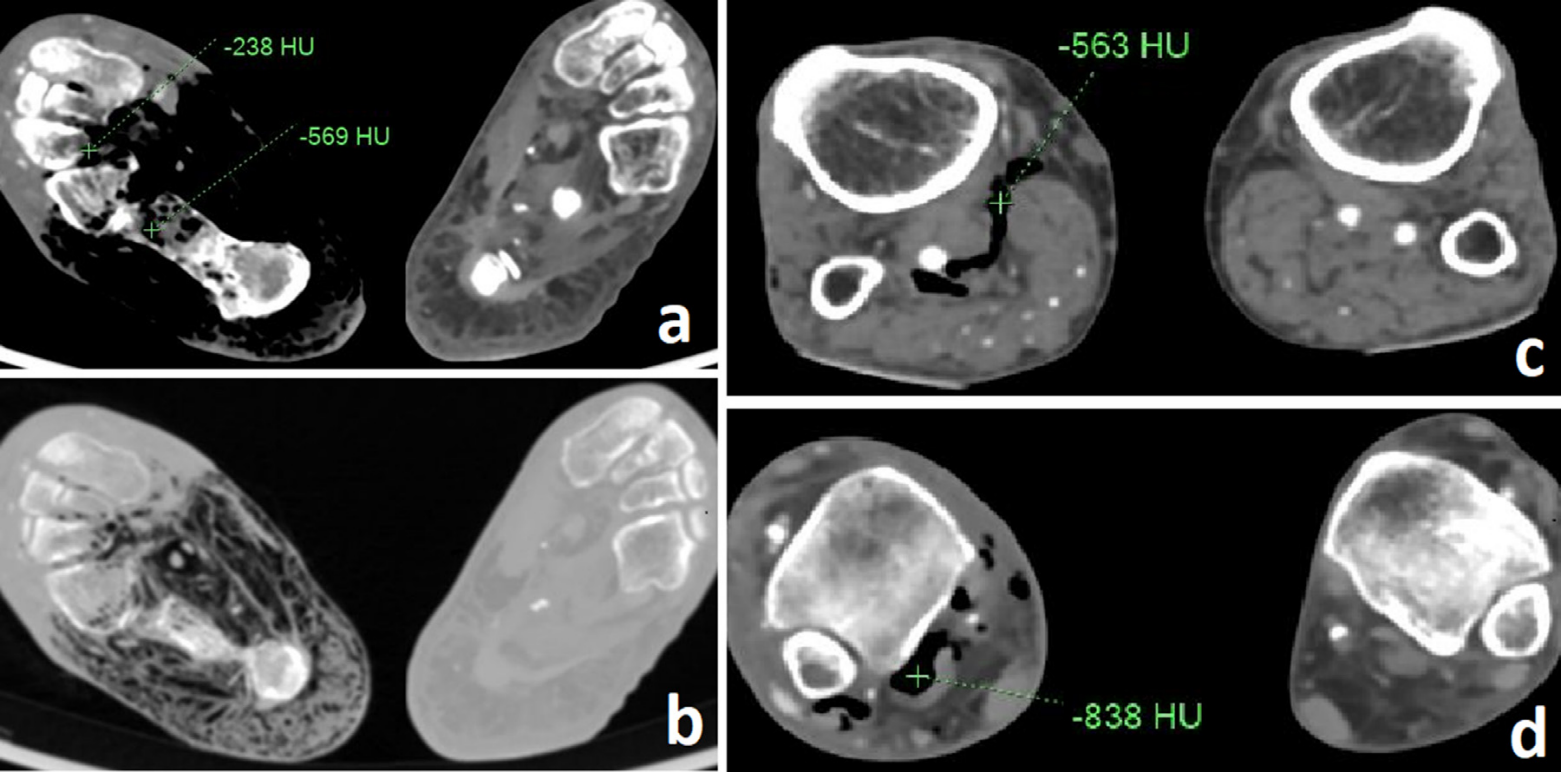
(A) Axial CT images of feet in soft tissue window of a 59-year-old male show cortical erosions and bone destruction in tarsal bones of right foot (calcaneus, cuboid and cuneiforms) with presence of intraosseous gas (indicated by Hounsfield units of air) and gross amount of gas in sub-cutaneous planes of right mid and hind foot. (B) Axial CT image in lung window supports the presence of air as black areas in the involved regions of the right foot. These features are suggestive of “Emphysematous osteomyelitis” involving tarsal bones of right foot with extension to soft tissues. (C and D) Axial CT angiogram images at the level just below knee (C) and at the level just above ankle (D) show presence of gas in deep tissue plane of right leg likely due to tracking up of air/infection from foot; visualized muscles in right leg show more or less normal bulk compared to that on left side. CT angiogram images in C and D also demonstrate normally opacified arteries of the leg without obstruction/thrombosis supporting the infective etiology rather than incited by vascular cause.
